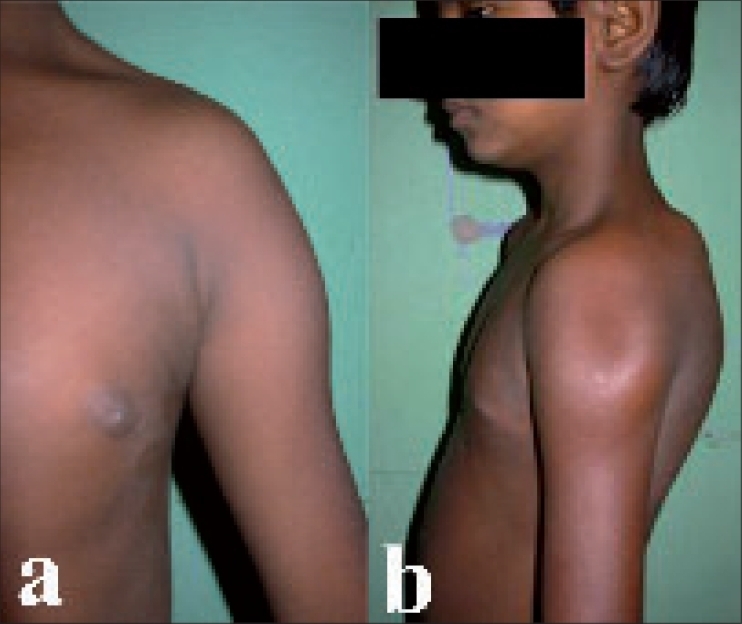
Preoperative clinical photographs of Case 3 showing abduction contracture of shoulder (a), with visible furrow overlying the contracture (b). The radiographs of this patient were normal
